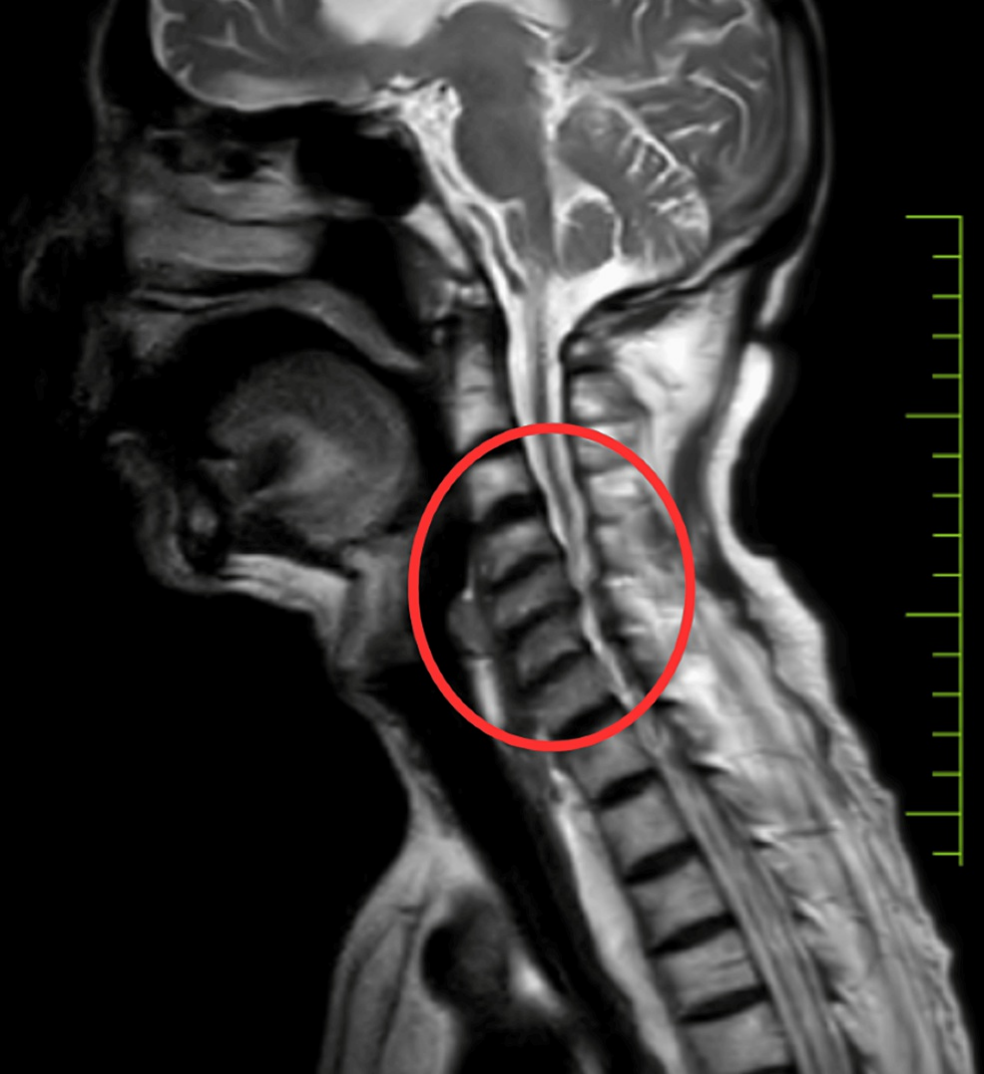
MRI of the cervical spine The circle shows C4-C6 parenchymal disc bulge indenting over the anterior thecal sac causing narrowing of neural foramen, and C6-C7 level posterior diffuse bulge indenting over the anterior thecal sac MRI: magnetic resonance imaging
